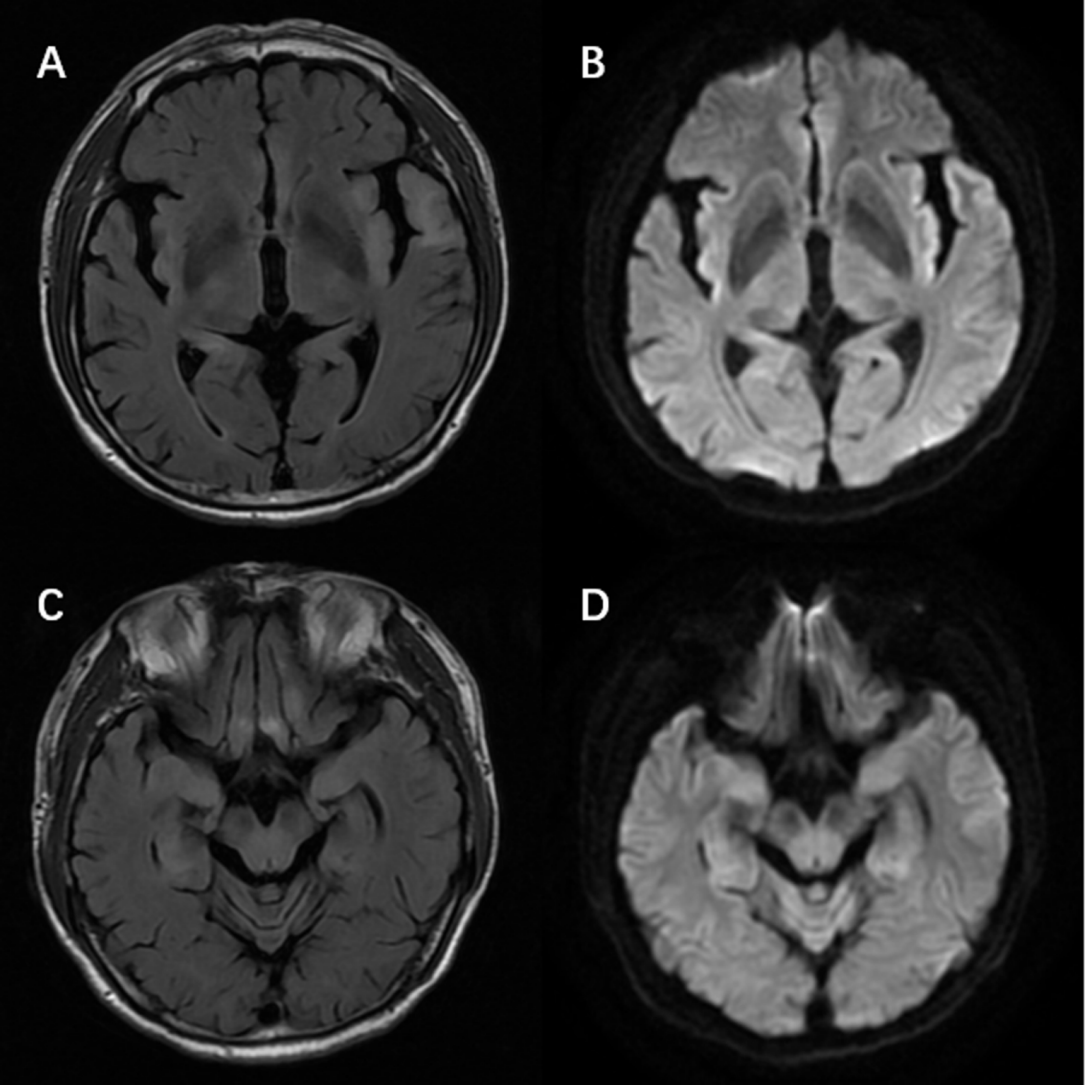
Magnetic resonance imaging of a patient with anti-alpha-amino-3-hydroxy-5-methyl-4-isoxazolepropionic acid receptor encephalitis showed T2/fluid-attenuated inversion-recovery sequency hyperintensity in left temporal lobe, bilateral hippocampus, frontal lobes, insula (A, C) with restricted diffusion on diffusion weight imaging at the corresponding region (B, D).
